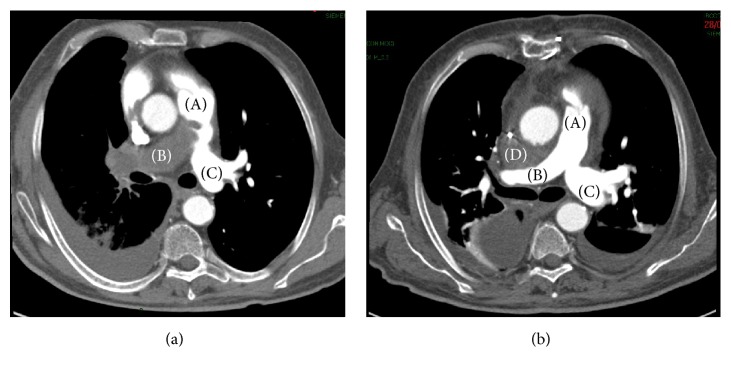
CT scan of patient number 10*. (a) Presurgical CT scan: right pulmonary artery completely occluded by soft tissue mass (B); proximal aspect of the mass in the pulmonary trunk (A); left pulmonary artery appears preserved (C). (b) Postsurgical CT scan (same level): the intra-arterial mass has been completely removed. Small mediastinal haematoma (D) between ascending aorta and right pulmonary artery.
